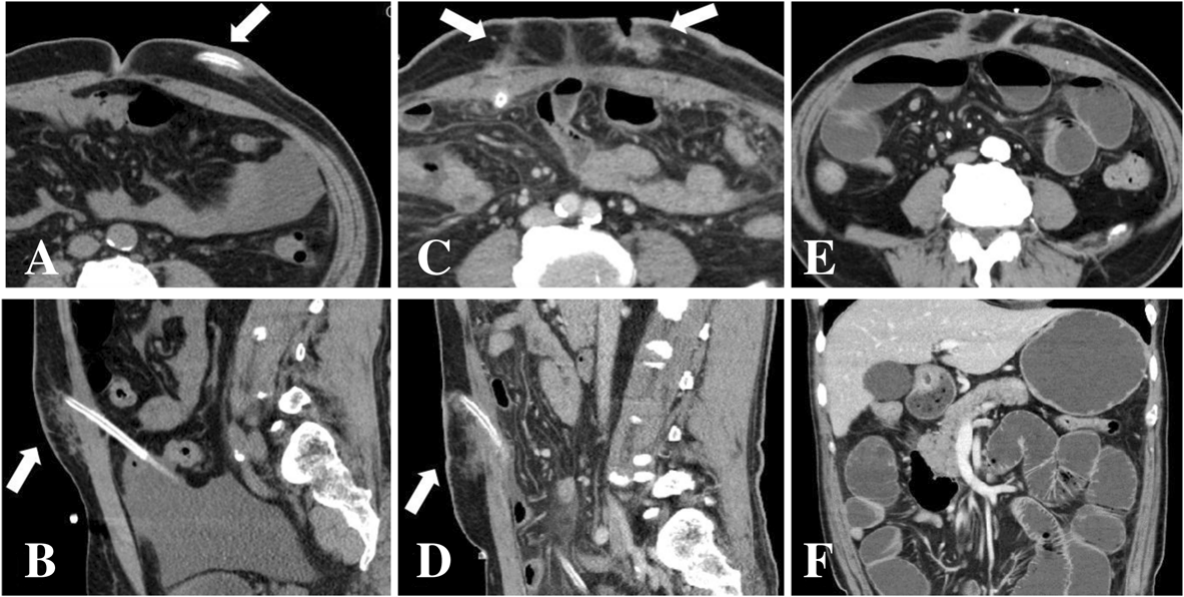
Radiological findings. On day 7, abdominal computed tomography revealed an increased density of fatty tissue around the PD catheter (arrows in a, b). On day 30, wound dehiscence was observed at the original exit site, with increased density of fatty tissue around the new catheter (arrows in c, d). On day 101, intestinal dilation was observed (e, f)
